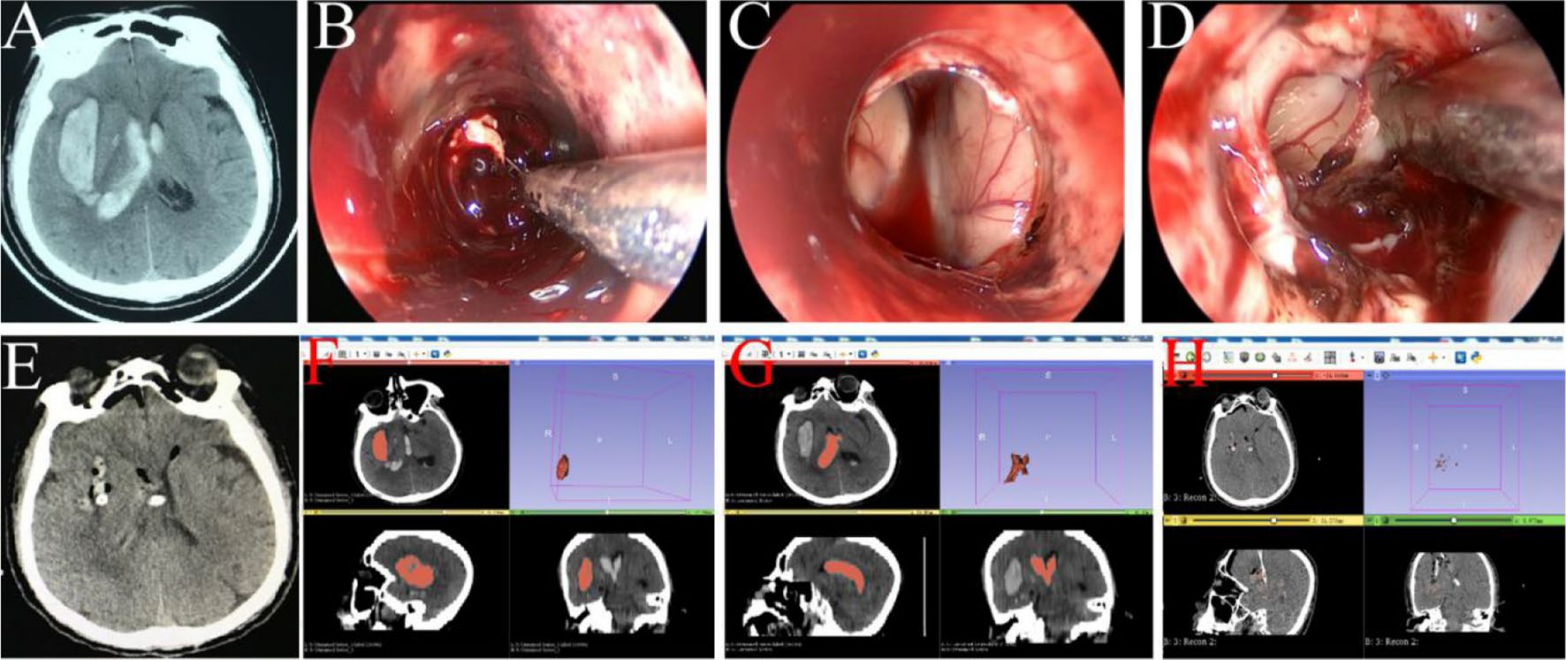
A right basal ganglia hemorrhage with extension into the ventricles was evacuated by transcranial neuroendoscopy. (A) CT before the operation showed a hematoma located in the right basal ganglia with extension into the ventricles. (B) The right basal ganglia hematoma was removed. (C) The hematoma in the right lateral ventricle was evacuated. (D) The hematoma in the left lateral ventricle was evacuated under neuroendoscopy through a transparent septum. (E) Postoperative CT showed satisfactory hematoma evacuation. (F) The preoperative volume of the hematoma in the right basal ganglia was 20.4 ml. (G) The preoperative volume of the hematoma in the ventricles was 21.7 ml. Thus, the total hematoma volume was 42.1 ml before the operation. (H) The postoperative hematoma volume was 1.41 ml, and the evacuation rate was 96.7%.
